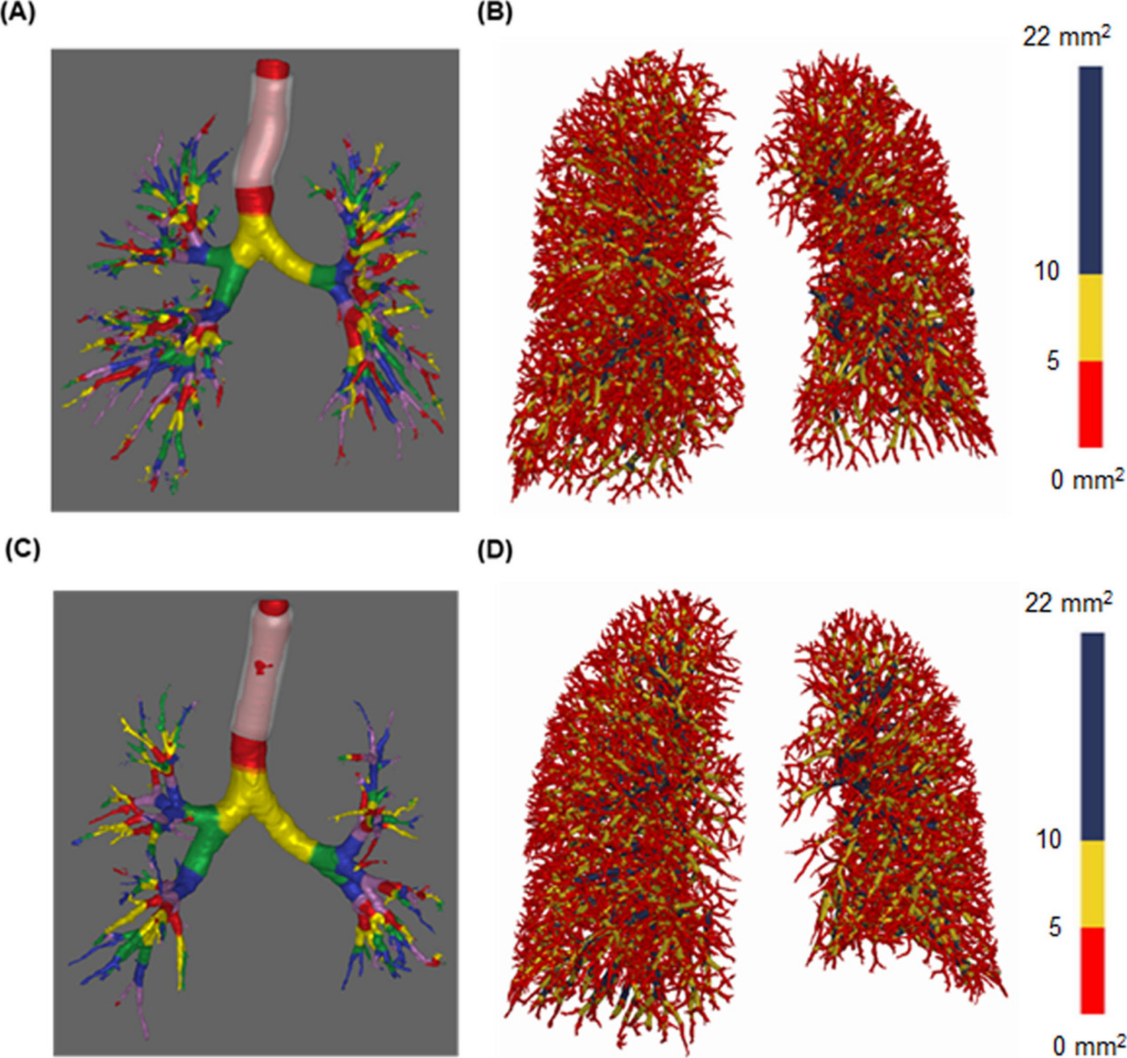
CT images of a patient with high AVR and of one with low AVR. Three dimensional images of the airways and lung vessels in patients with high AVR (A, B) and low AVR (C, D). The prolongation of the airway tree is observed in the patient with high AVR, while a less complex airway tree is observed in the patient with low AVR. (A, B) 75 years old, Male. AVR: 0.411, TAV/LV: 0.024, TBV/LV: 0.059, TAC: 332. (C, D) 63 years old, Male. AVR: 0.160, TAV/LV: 0.009, TBV/LV: 0.058, TAC: 238. AVR, volume ratio of the airway to lung blood vessels; CT, computed tomography; LV, lung volume; TAV, total airway luminal volume; TBV, total blood vessel volume; TAC, total airway count.
